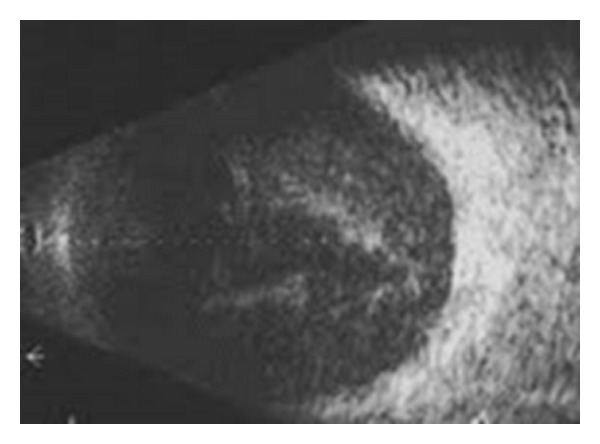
Ultrasonography OD—diffuse vitreous condensation.
